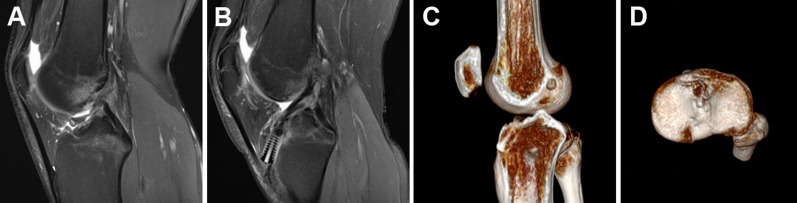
Radiological examination of STSB ACL reconstruction. A Preoperative MRI showed ACL rupture. B MRI at the last follow-up showed complete graft healing. C 3D CT showed the femoral socket and the tibial tunnel. D 3D CT showed the tibial socket
